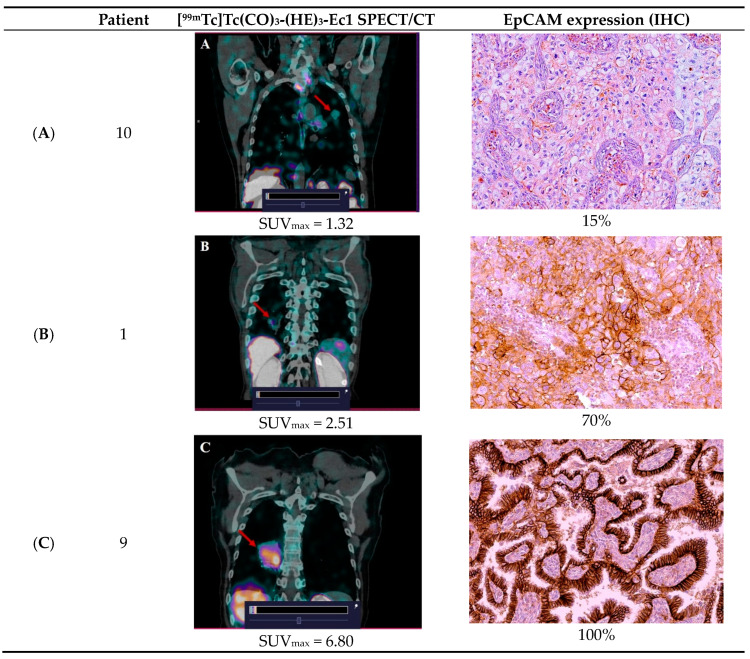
Comparison of SPECT/CT with [^99m^Tc]Tc(CO)_3_-(HE)_3_-Ec1 and EpCAM expression level in lung cancer patients. (A) SUV_max_ = 1.32 and EpCAM expression level—15%. (B) SUV_max_ = 2.51 and EpCAM expression level—70%. (C) SUV_max_ = 6.80 and EpCAM expression level—100%.
